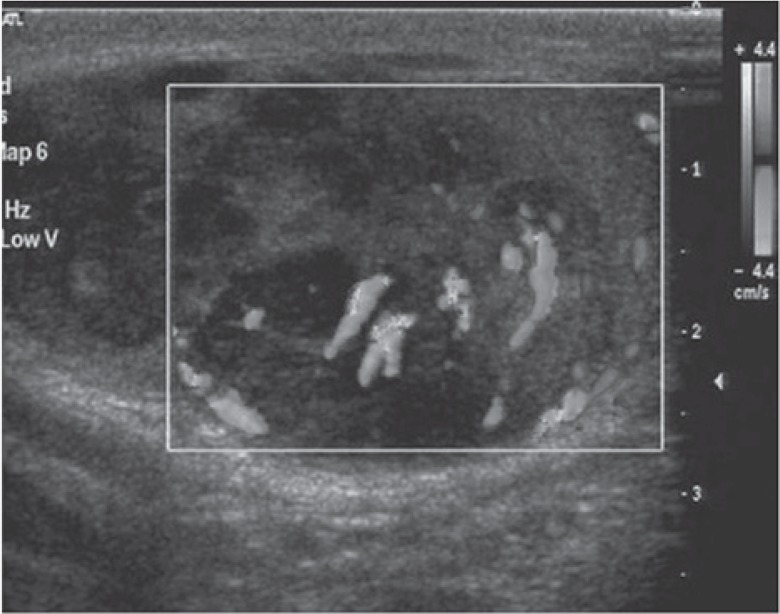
Doppler sonography image of the right testicular region demonstrating a large mass with mixed echogenicity and blood flow signal.
